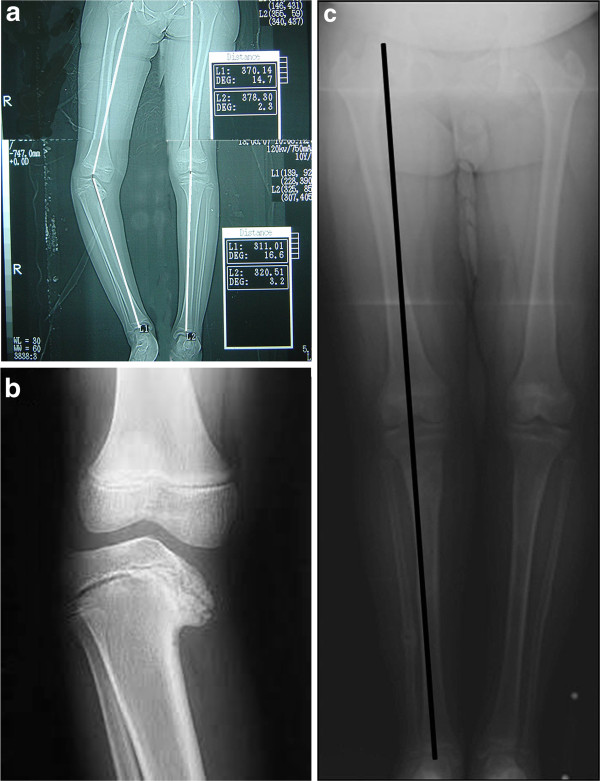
(a) Preoperative A-P radiogram of a 12-year-old female with right sided late onset tibia vara with a preoperative DA angle of −45°. (b) Roentgenogram of the right knee shows stage IV of the Langenskiold staging. (c) follow-up A-P radiogram after 4 years shows no recurrence.
